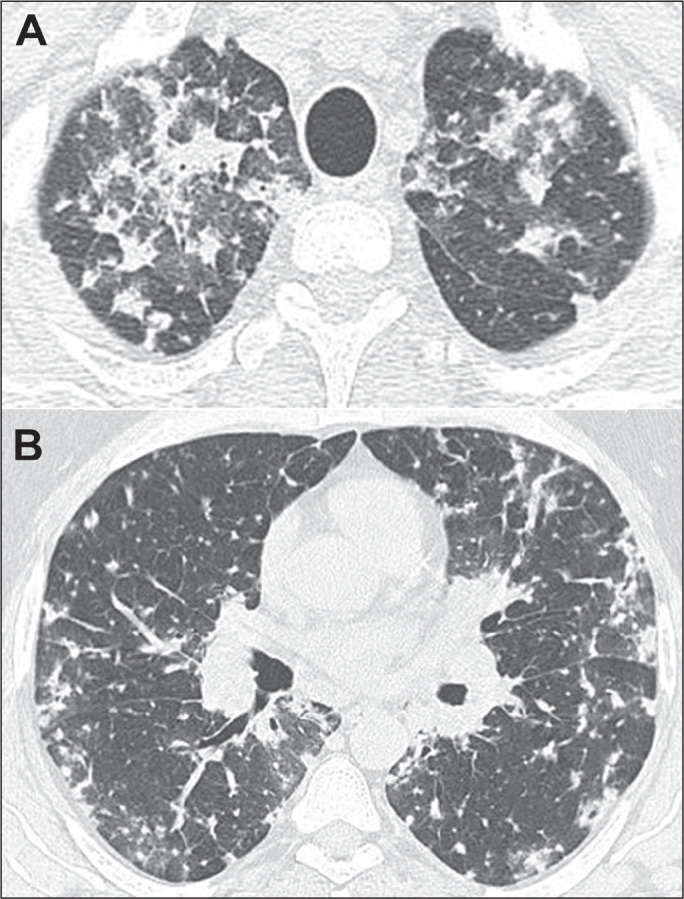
CT of a 57-year-old man with positive serology, showing disseminated
